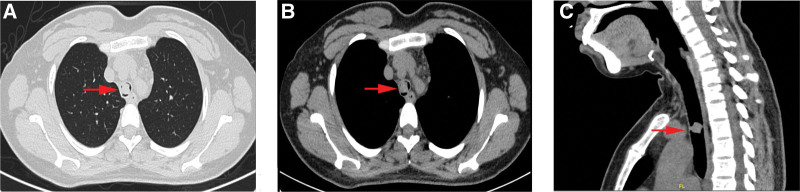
Computed tomography (CT) scan of the chest. Enhanced CT scans revealed a soft tissue density nodule shadow in the tracheal cavity. The size of the lesion was about 1.3 × 1.2 cm with a CT value of about 57 HU. The tracheal lumen was narrow and the tracheal ring was complete. The remaining bronchi were unobstructed, without expansion or stenosis. CT = computed tomography.
